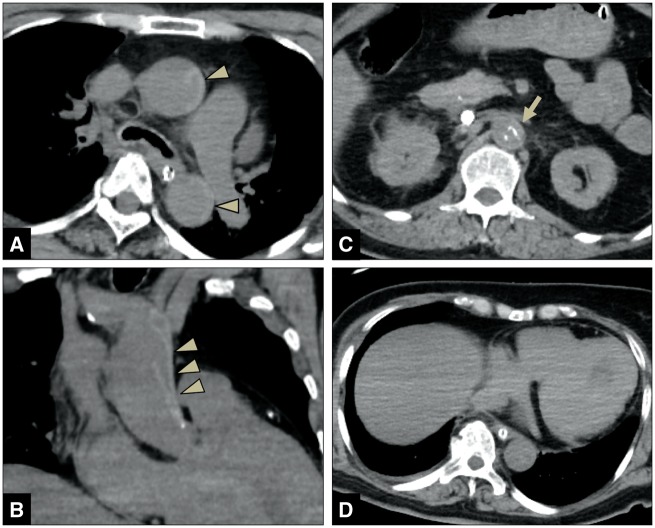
Computed tomography imaging of Patient 3 shows aortic dissection extending from the root of the ascending aorta to the common iliac artery. The true lumen from the ascending aorta to the descending thoracic aorta completely collapsed due to the enlarged false lumen (*A*/*B*-arrowheads) and inward shift of the calcified intima at the abdominal aorta (*C*-arrow). No findings, such as bloody pericardial effusion and haemothorax, suggesting a rupture of the aorta (*D*).
